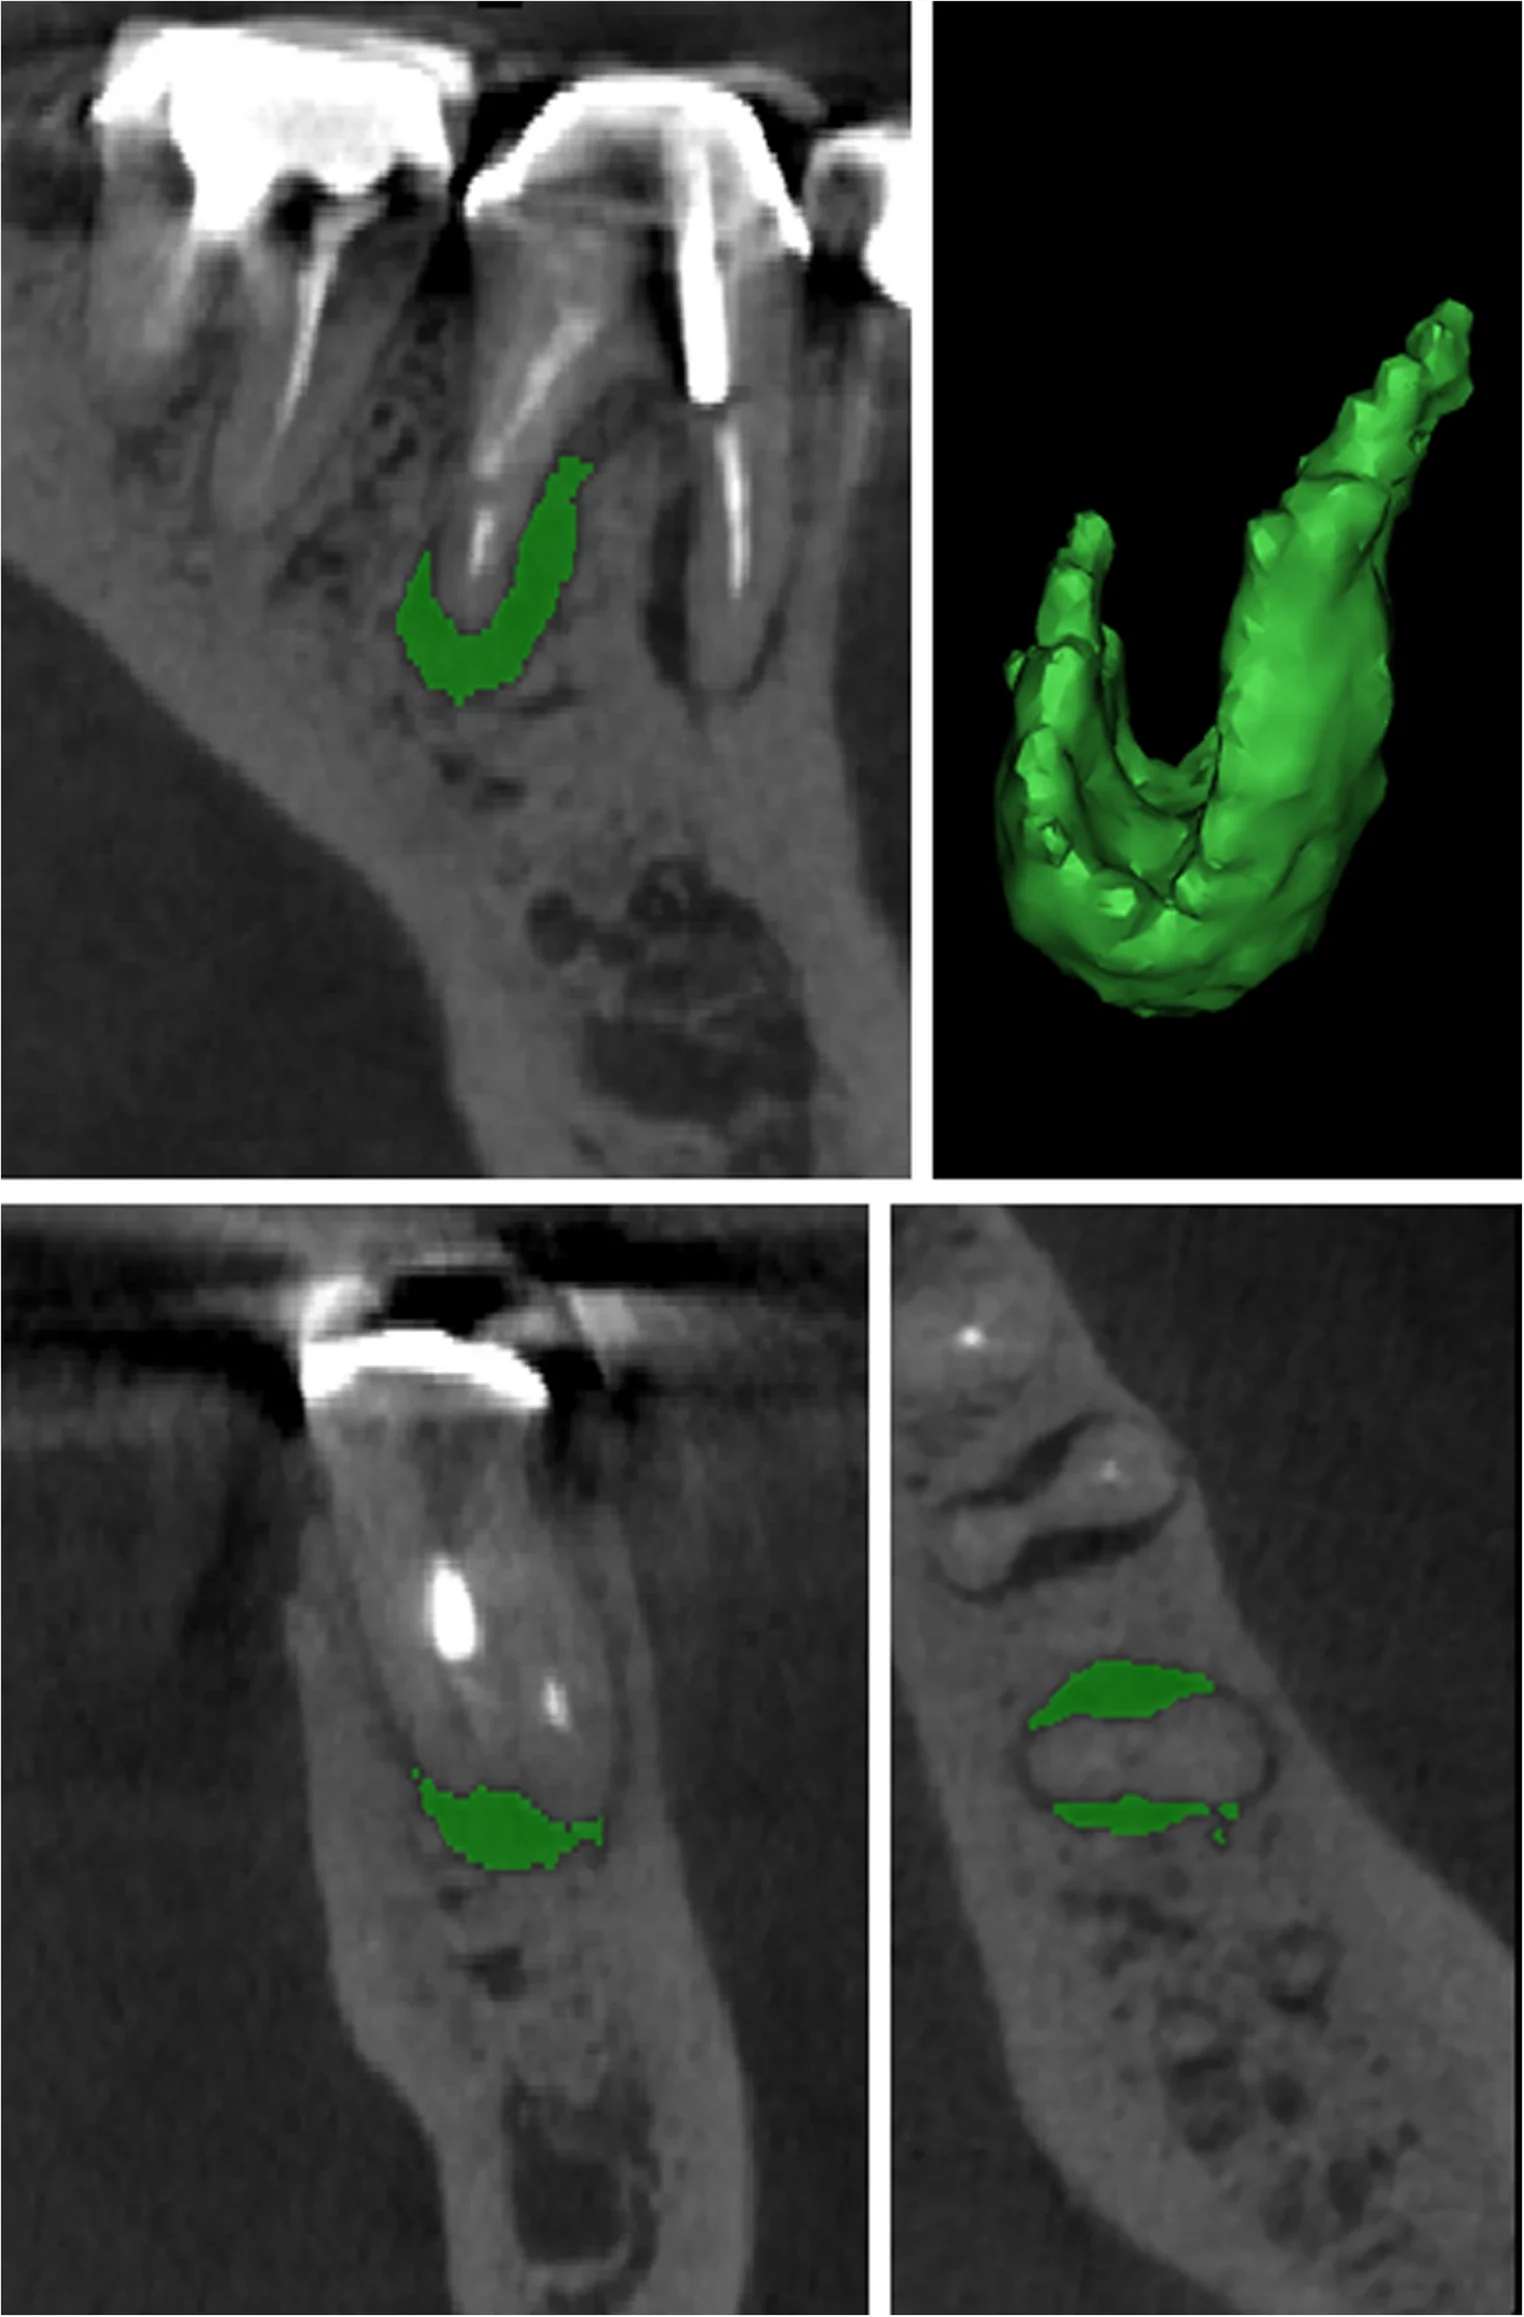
Three-dimensional reconstruction of an apical periodontitis lesion from CBCT scan
